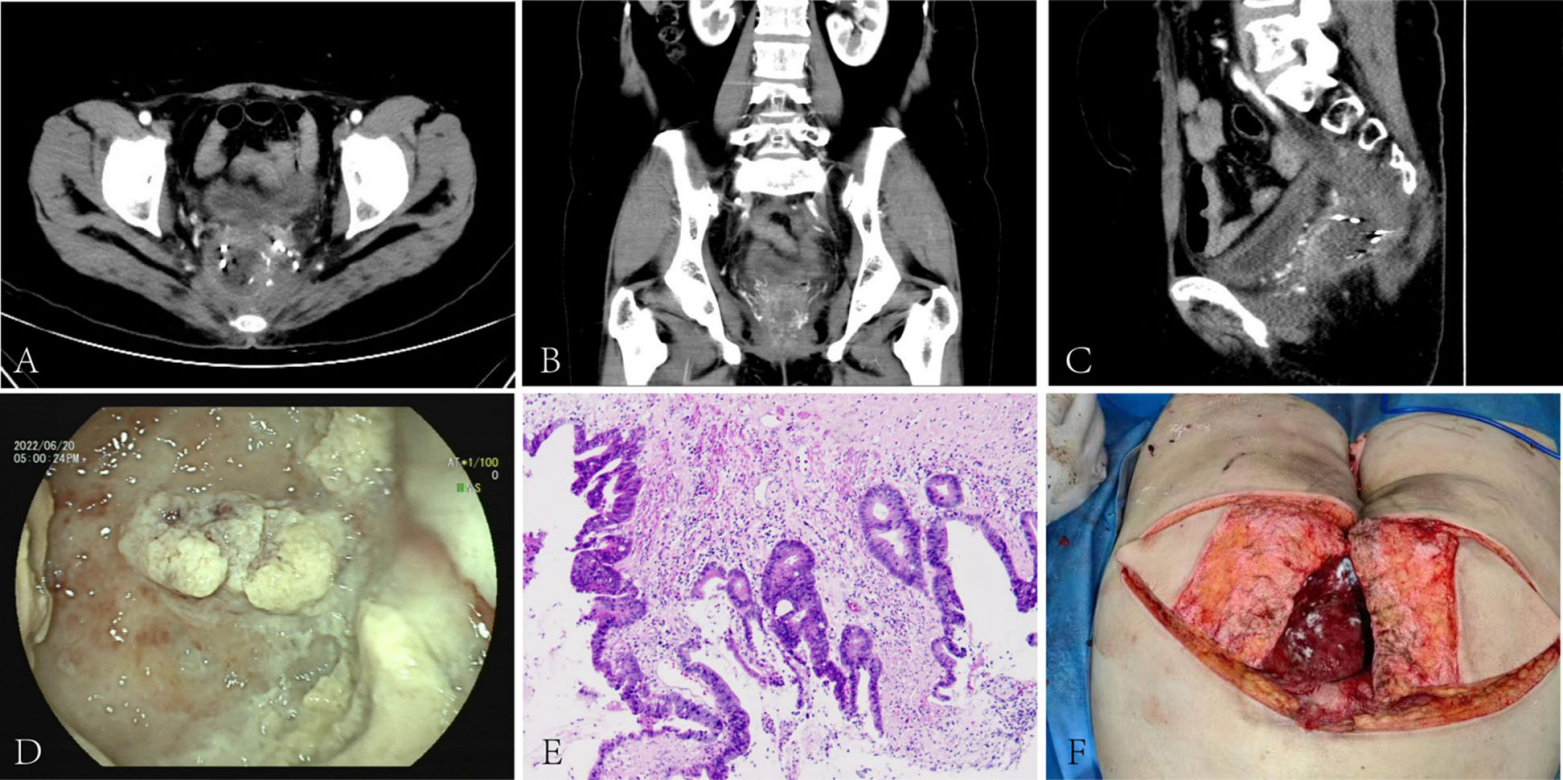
Pre-pelvic exenteration colonoscopy, CT, post-operative pathology and reconstruction: CT scan showed a mass-like slightly hypodense shadow next to the right side of the rectal stump. The lesion was poorly demarcated from the right vaginal wall and presacral soft tissue and invaded the surrounding tethered fascia and right levator ani muscle. The rectal cavity was compressed and deformed(A-C). The blind end was observed at 8cm away from the anus through the natural anus, and the intestinal mucosa was covered with white contents(D). The cancer tissue invaded the entire bowel wall, the entire vagina, involving the surrounding striated muscle tissue and adipose tissue, the local prolusion of the bladder mucosa, and localized necrosis of the serous layer and the muscular layer(E). After pelvic exenteration, the patient underwent free myocutaneous flap graft (F).
